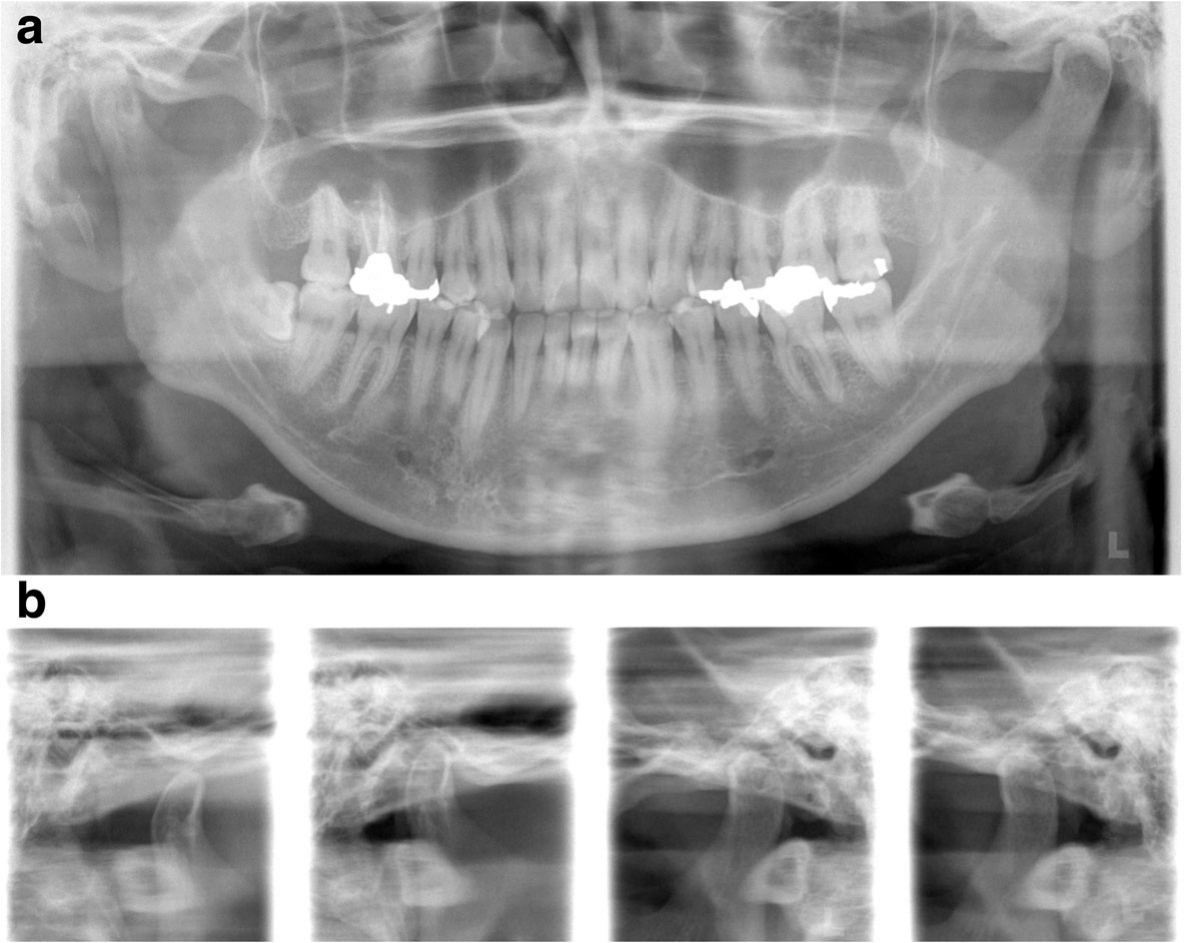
Conventional radiographs. a Panoramic radiography showing no particular findings relevant to the symptoms. b Panoramic temporomandibular joint projection method demonstrating slight restriction of jaw movement on the left
